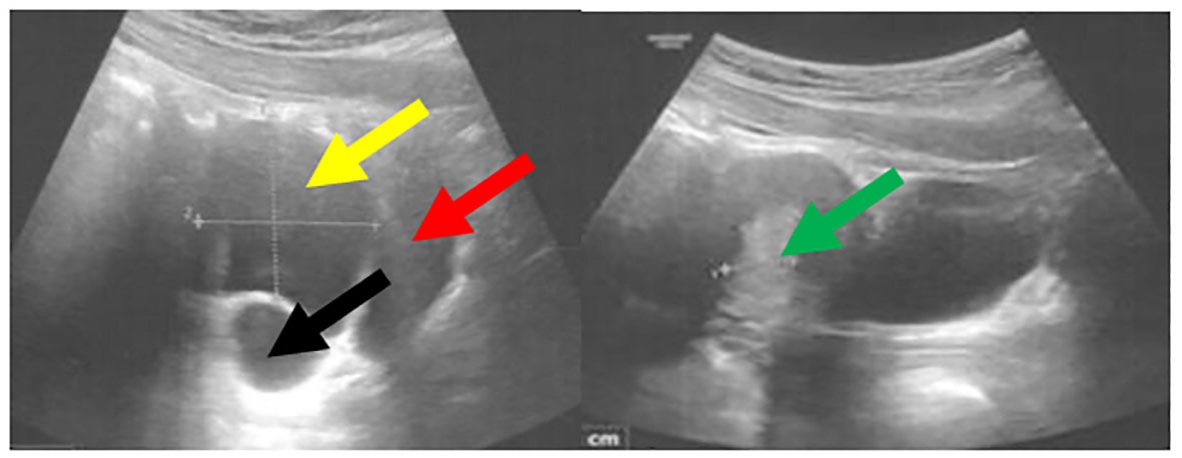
Pelvic ultrasound performed at 13 years and 8 months of age demonstrated a cyst within the right ovary measuring 5 × 4 cm. The bladder is indicated by the red arrow, the uterus with an endometrial thickness of 13.3 mm by the green arrow, the cyst by the yellow arrow, and the right ovary by the black arrow.
